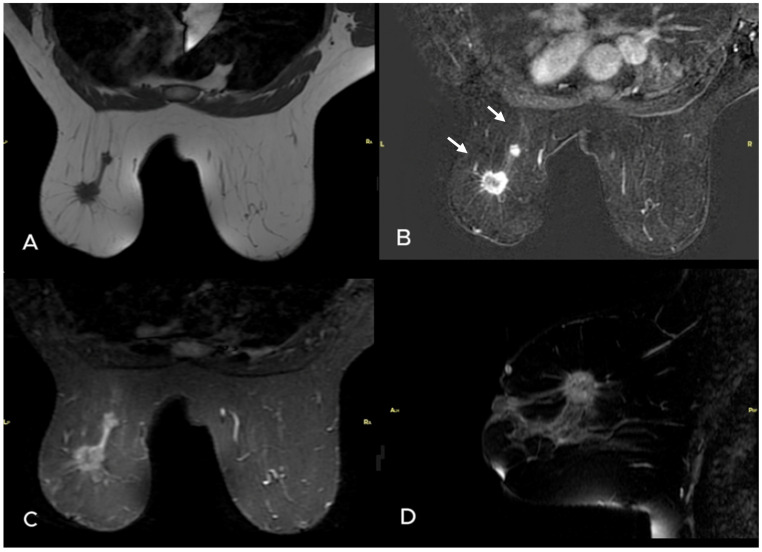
A 38-year-old woman presented with nipple retraction in the left breast. Unenhanced (A) and subtracted (B) MR images revealed 2 masses. The largest had spiculated margins and demonstrated peripheral enhancement, while the second one was well circumscribed and lobulated and demonstrated homogeneous enhancement (white arrows). Axial and sagittal TSE STIR (C,D) images showed no significant edema. Core needle biopsy results of both masses were invasive ductal arcinoma, and ER was 70%, PR was 95%, HER2 was 2 (+) (FISH (−)), and Ki-67 index was 20% for the larger mass. Postoperative pathology revealed 2 metastatic lymph nodes in the axilla.
